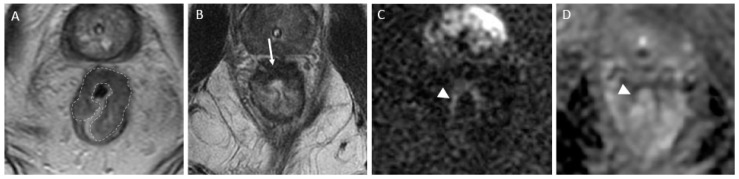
Near-complete response in a 65-year-old man with middle rectal adenocarcinoma. (A) Baseline axial T2-weighted MR image shows an intermediate-signal-intensity, near-circumferential low rectal tumor (dotted line). (B) Axial T2-weighted MR image after the completion of neoadjuvant chemoradiotherapy shows a slight response with a small amount of fibrosis and residual tumor signal intensity (arrow). Axial diffusion-weighted image (C) shows high signal intensity (arrowhead), and the axial apparent diffusion coefficient map (D) shows a corresponding low signal intensity (arrowhead), in keeping with restricted diffusion at the nonfibrotic portion of the tumor.
